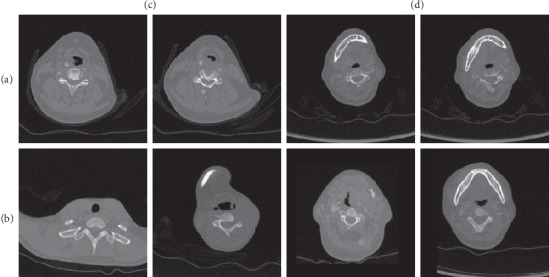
Samples of the multicenter CT images used for network training: (a) benign cases; (b) malignant cases; (c) plain CT scans; (d) enhanced CT scans.
